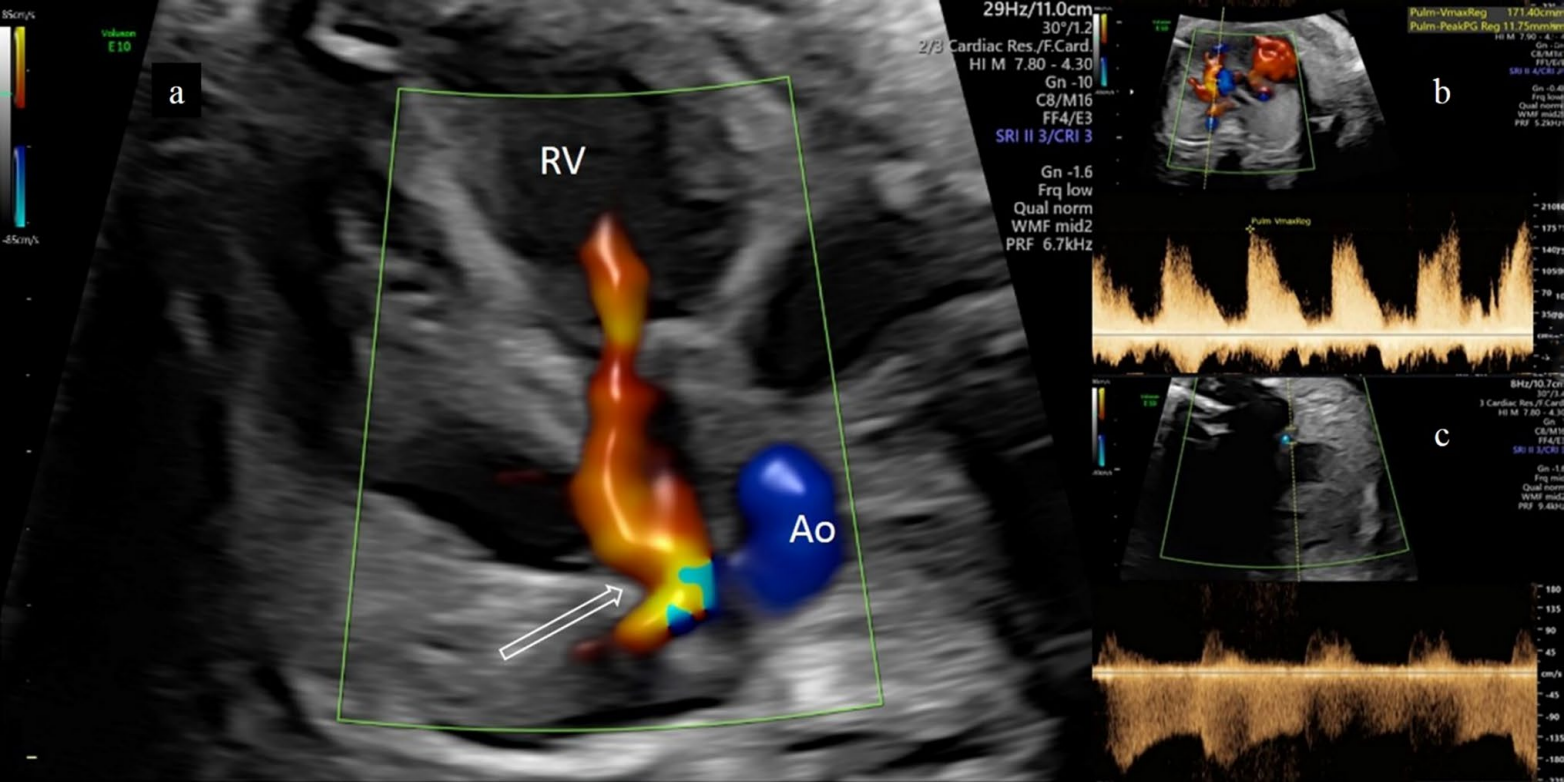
Case 1 a Color Doppler during systole at the four chamber view in a fetus with a severe Ebstein anomaly demonstrating a patent ductus arteriosus with a retrograde flow (open arrow) at 31 + 6 weeks gestation. b Severe pulmonary regurgitation with a peak velocity of 240 cm/s is shown, leading to a diagnosis of a circular shunt. c After 8 days of intrauterine treatment with indomethacin, constriction of a DA is demonstrated on color Doppler with an increase in the diastolic portion of the still reversed blood flow, as well as a peak systolic velocity of > 220 cm/s and distinctly increased diastolic flow with a PI of 0.68
